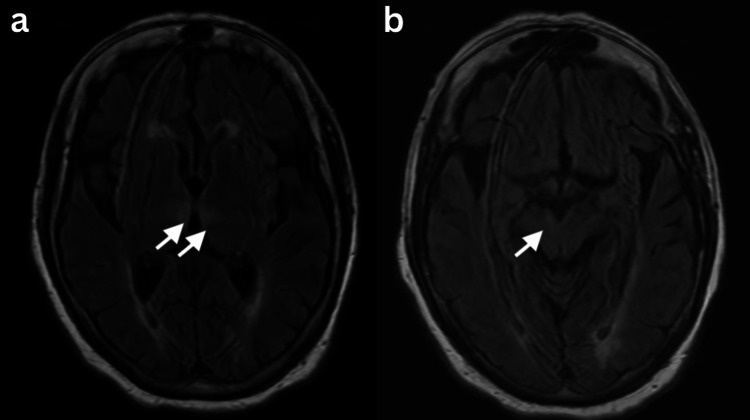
MRI fluid-attenuated inversion recovery (MRI-FLAIR) a) Bilateral thalamic and b) Right-sided rostral midbrain hyperdensity.
